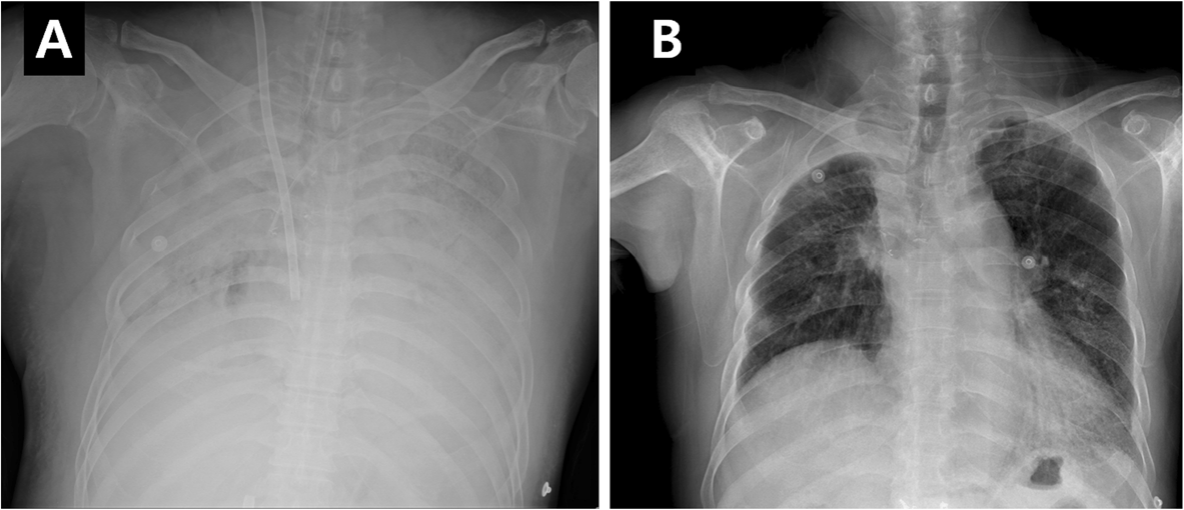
Conduction of extracorporeal membrane oxygenation (ECMO). Chest radiography shows diffuse pulmonary consolidations and a return cannula into the right internal jugular vein for ECMO (A), and improved bilateral pulmonary infiltrations after removal of ECMO (B).
